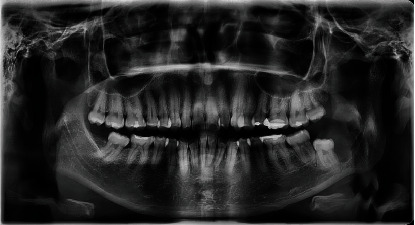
Orthopantomography image before Tooth #38 was extracted. Note the multicystic radiolucency on the left mandibular ramus.
